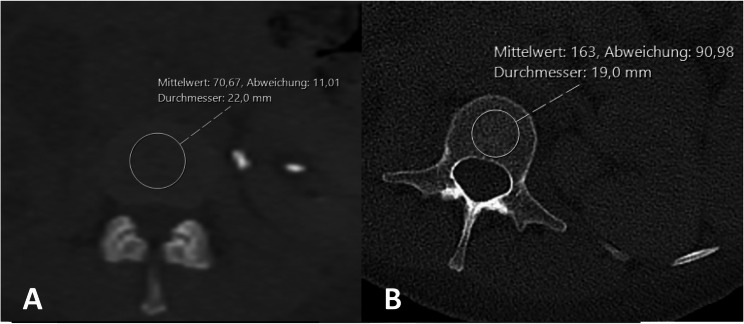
Measurement of the HU in the vertebral discs and the vertebral bodies. Axial reconstruction of a lumbar spine CT Scan. A circular region of interest was selected based on manually defined reference lines, having a diameter of app. 75% of the anteroposterior and transverse diameters of the vertebral body or the intervertebral disc. The average HU within the sample region was displayed by the software (Mittelwert). A shows exemplary a performed measurement in a vertebral disc – HU 70.67. B shows a performed measurement in a vertebral body – HU 163
